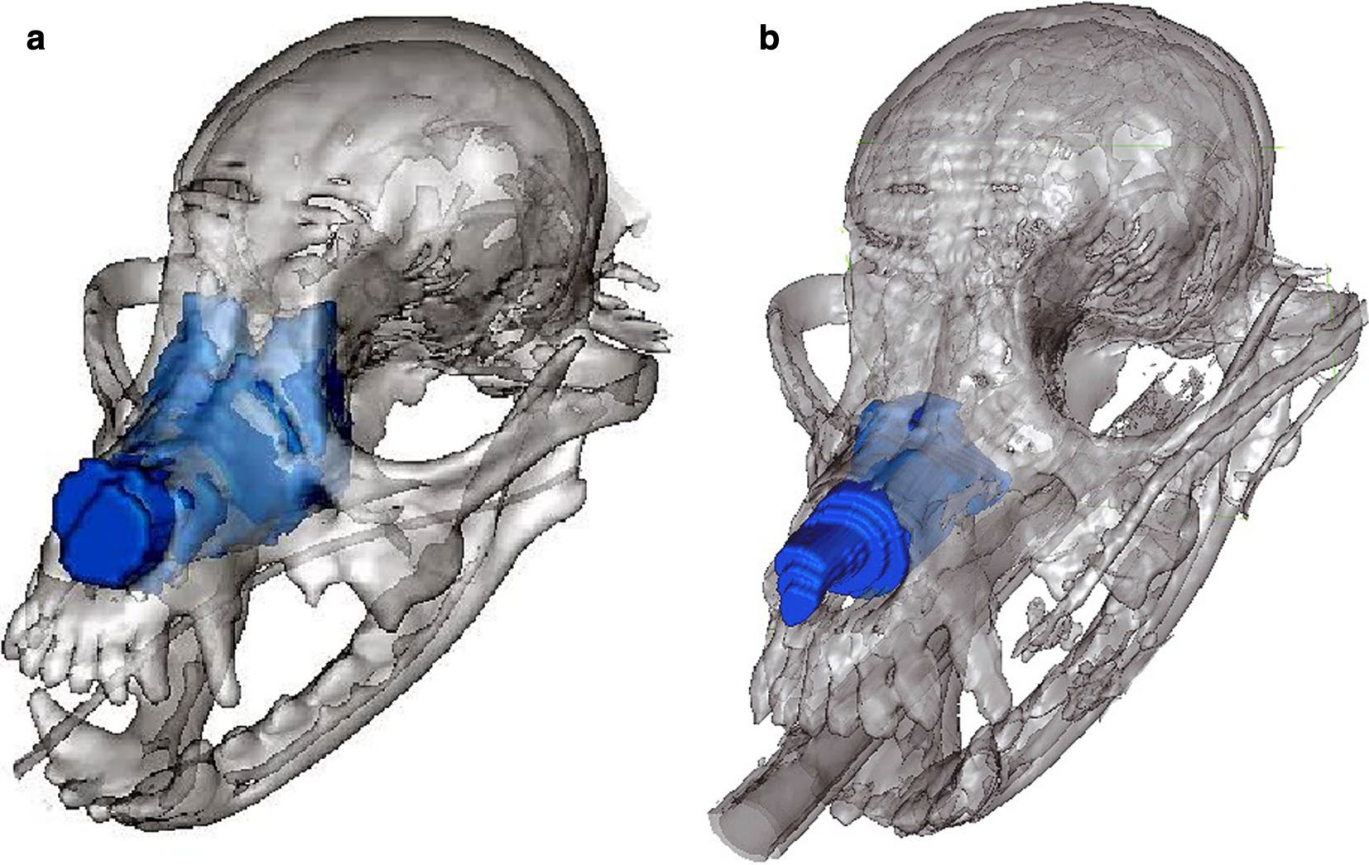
(Patient #7) a pre radiotherapy CT scan, b post radiotherapy CT scan (1 month post radiation therapy)
